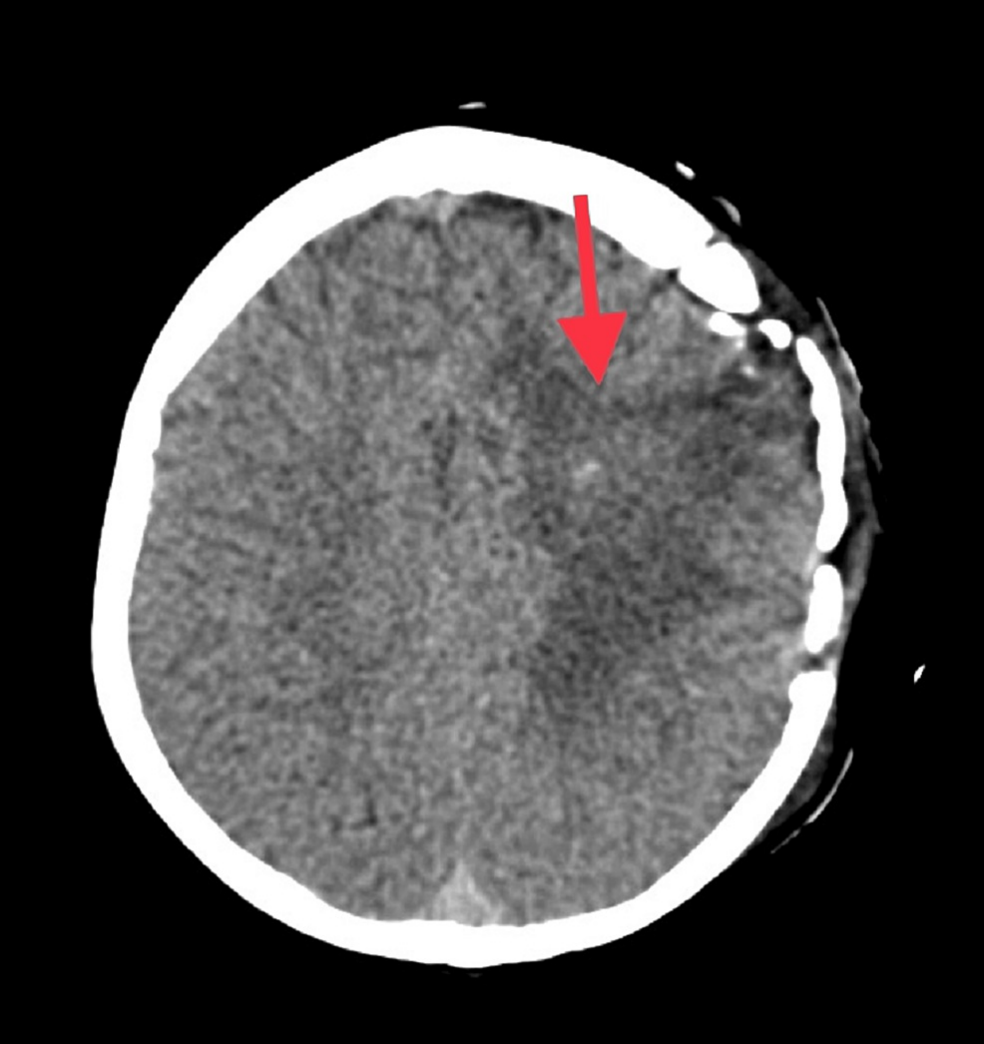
Axial non-contrast CT section showing post-operative oedema in the left frontoparietal lobe and adjacent post-operative calvarial defect
